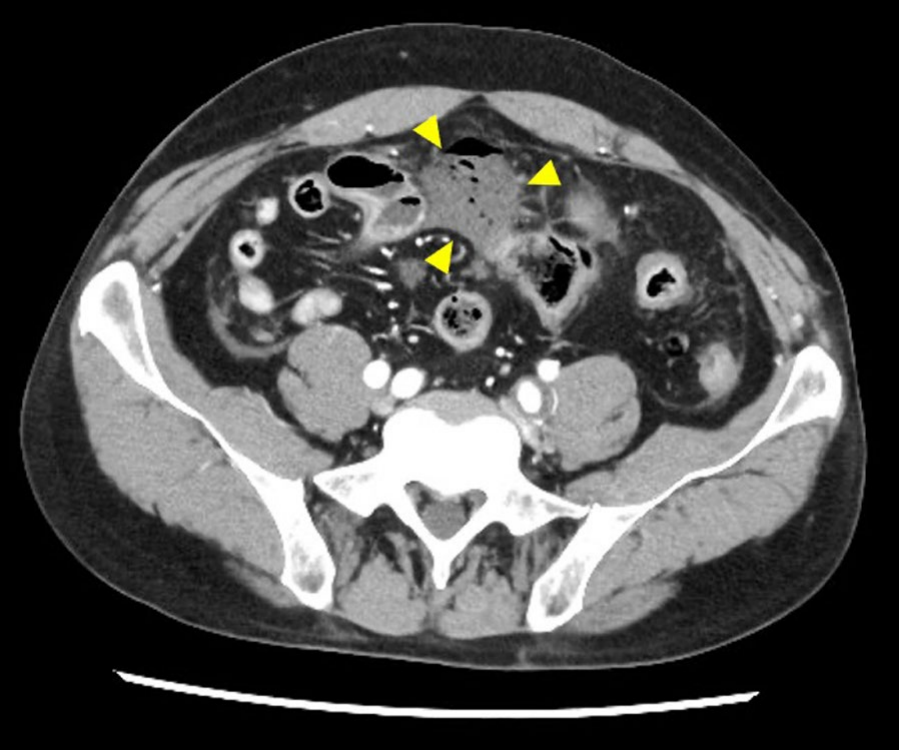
Preoperative abdominal CT findings. Fluid accumulation and free air around the sigmoid colon are observed
